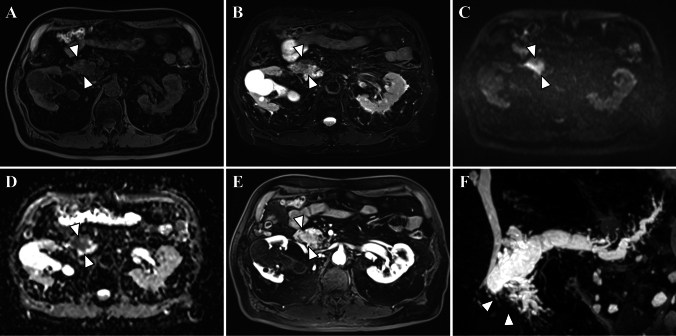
Magnetic resonance imaging (MRI) findings. MRI revealed a mass in the main pancreatic duct of the pancreatic head (arrowhead). The mass was recognized as a low-intensity area on T1-weighted imaging (T1WI) (A) and T2WI (B). It was hyperintense on diffusion-weighted images (DWI) (b = 800 s/mm^2^) (C) with decreased apparent diffusion coefficient (ADC) values (D). E The mass was not well contrasted on gadolinium-enhanced MRI (Gd-MRI). F On magnetic resonance cholangiopancreatography (MRCP), the main pancreatic duct was obstructed at the head of the pancreas (arrowhead). The dilation of the branch pancreatic ducts tail side to the obstruction was observed
